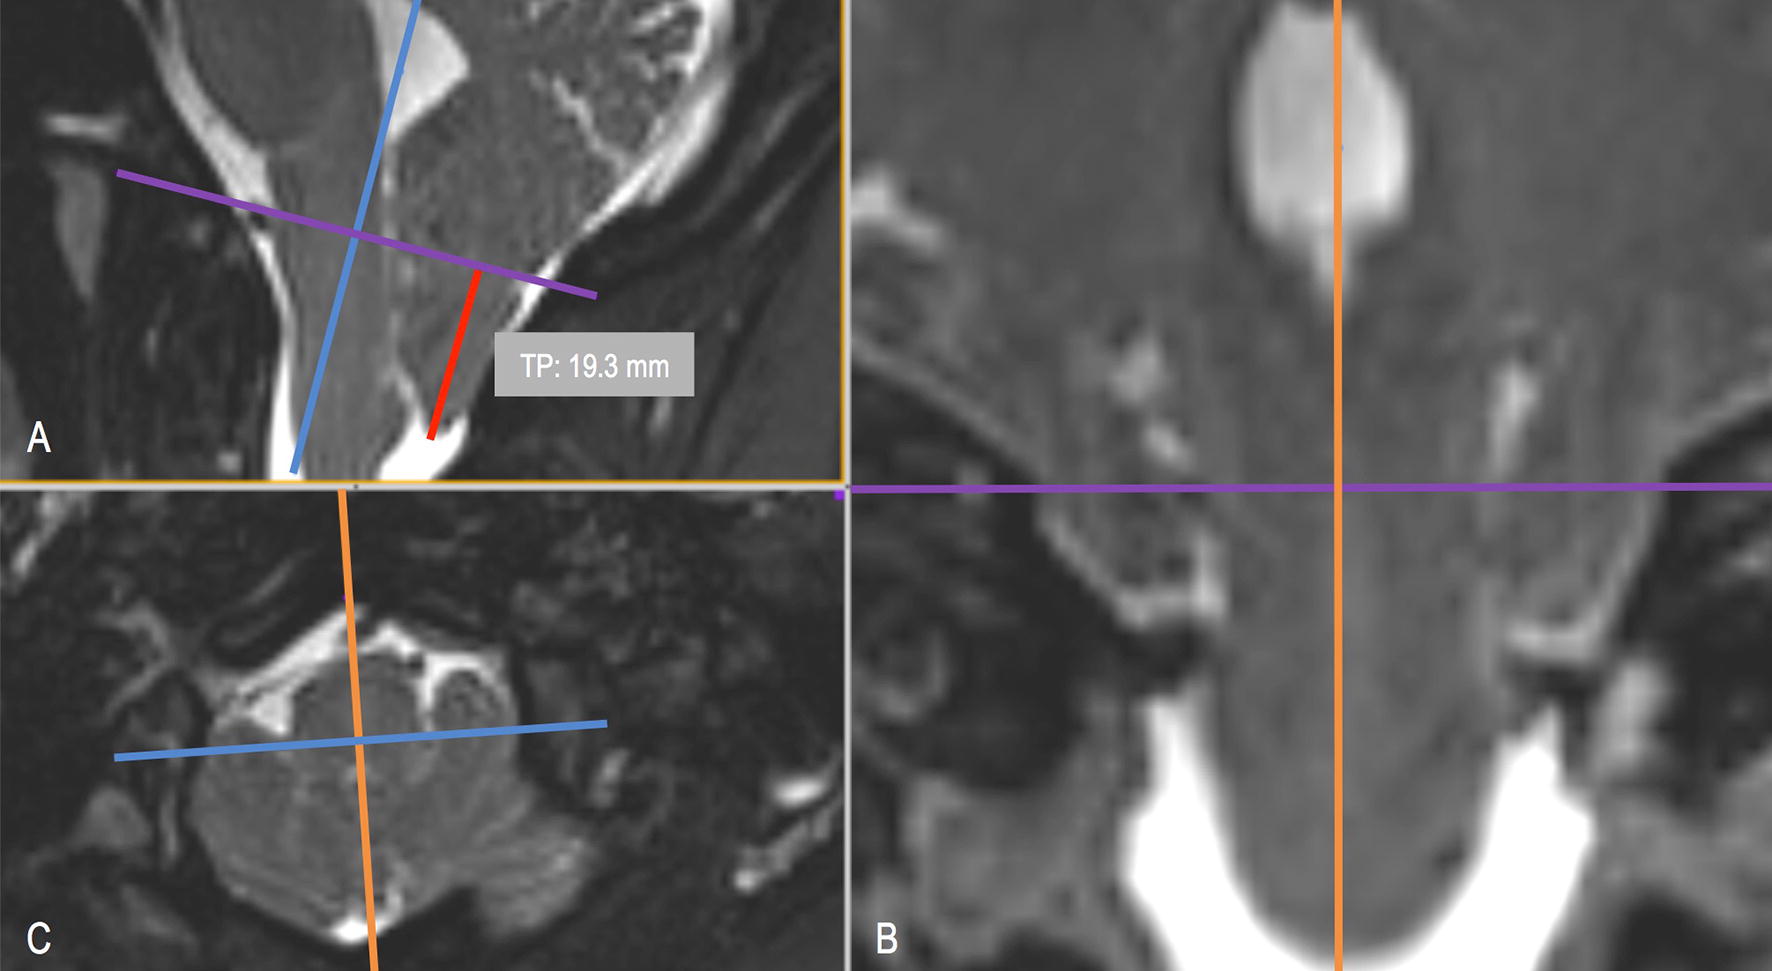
3D multi-planar reconstruction of tonsillar position (TP, red) morphometric measurement for a T2-weighted MRI of subject 27 in this study: A mid-sagittal plane with McRae’s line shown as a violet line. B Coronal plane with orientation of McRae’s line shown as violet line. C Axial plane visualization through McRae’s line showing partial obstruction of cerebrospinal fluid (CSF) space around the spinal cord
